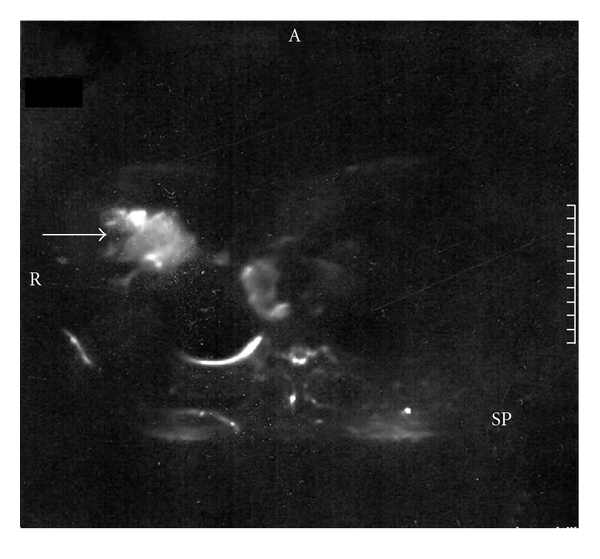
Axial stir (short-tau-invesion-recovery) MR image of the upper thorax demonstrates a lesion located in the right part of the anterior thoracic wall and shows increased inhomogeneous signal intensity. The lesion has irregular contour, involving the major pectoralis muscle. Inside the lesion, a node is noticed.
